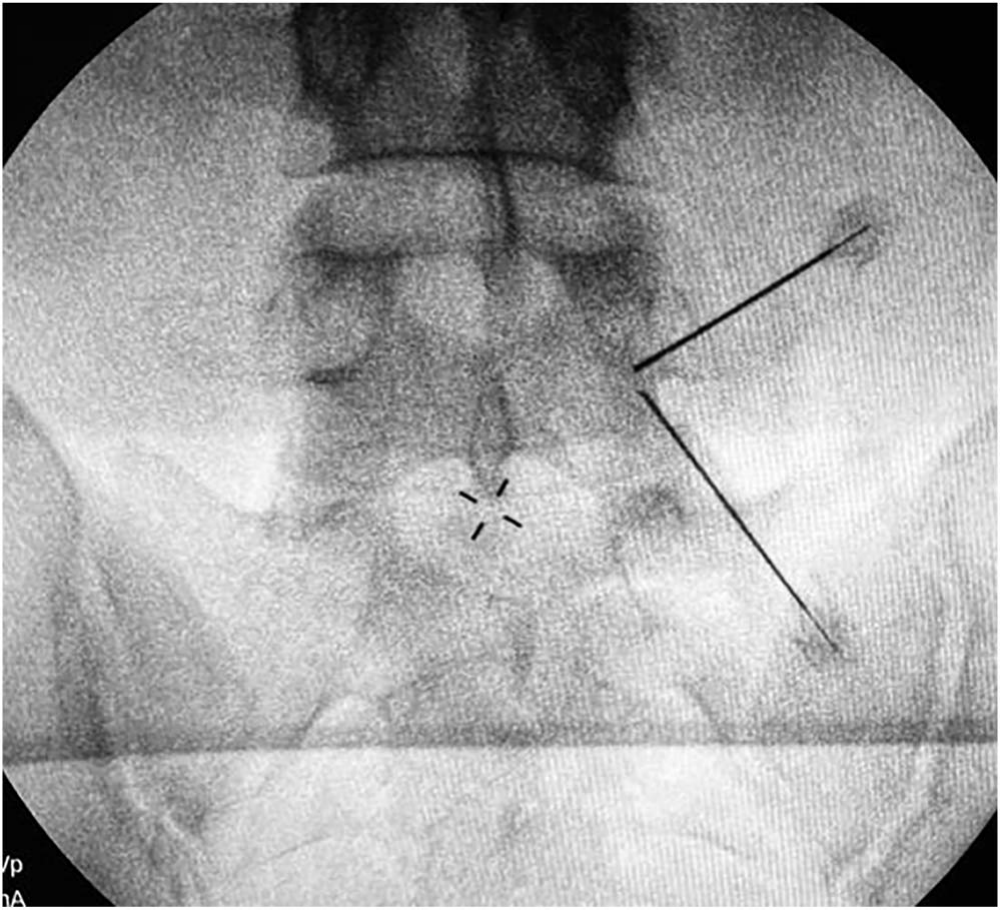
AP X-ray with needles to confirm proper docking point placement.
